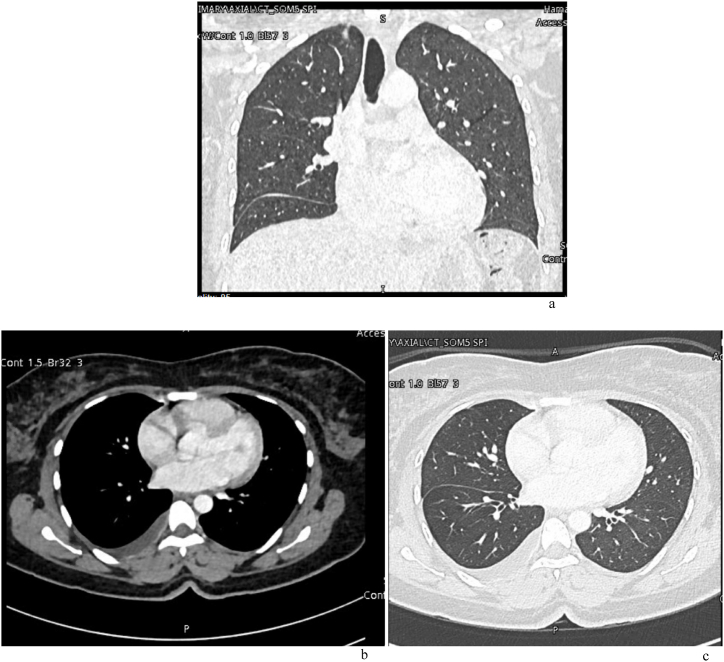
Chest CT scan done after discharge, showing right sided pleural effusion with subtle pleural thickening and bilateral apical thickening.
